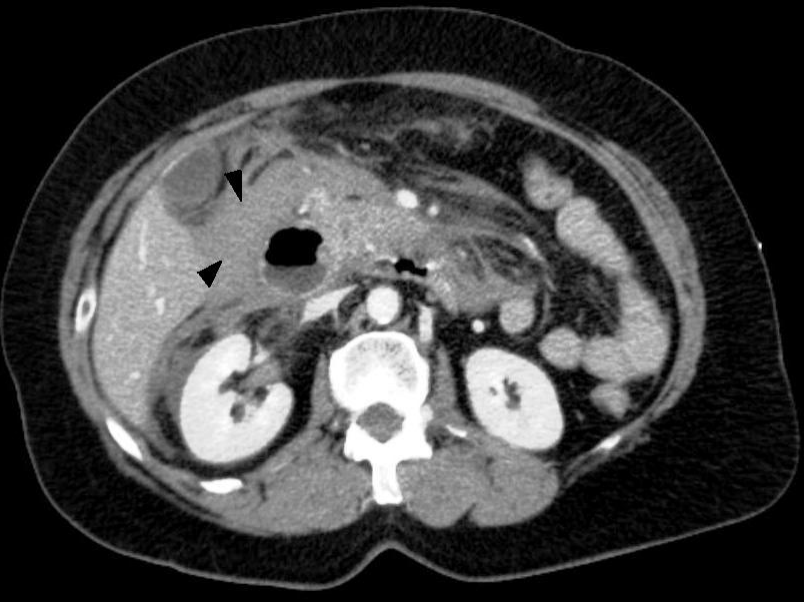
Abdominal CT (axial) with contrast demonstrated a large density around the duodenum, the fluid densities were felt to represent a hematoma. (Black arrowhead).
